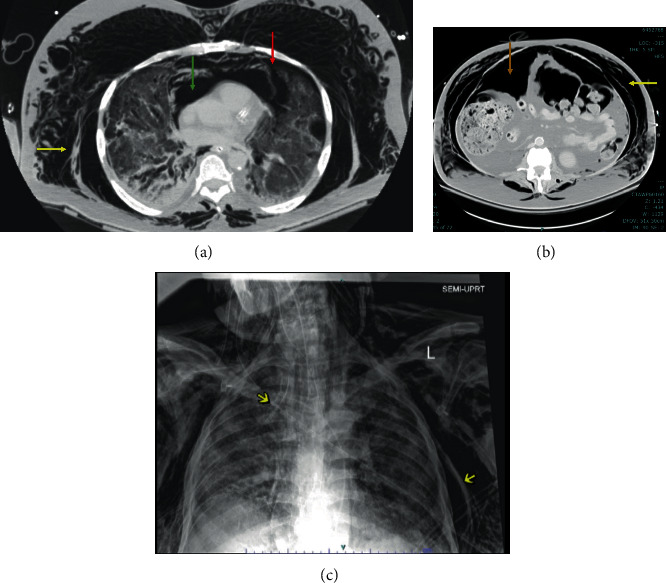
(a) CT of the chest showing moderate-sized left pneumothorax (red arrow), pneumomediastinum (green arrow), and extensive subcutaneous emphysema of the chest wall (yellow arrow). (b) Abdominal CT with large pneumoperitoneum (brown arrow) and subcutaneous emphysema of the abdominal wall (yellow arrow). (c) CXR demonstrating right-sided chest tube and subcutaneous emphysema of chest wall.
